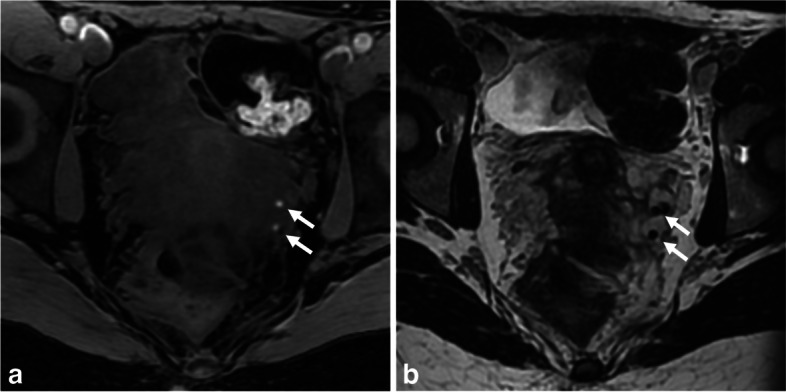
Parametrial left-sided phlebolith in an 18-year-old woman. a Axial T1-W and (b) axial T2-W MR images show a punctiform T1-hyperintense “spot” matching a T2 “dark spot” within the lumen of a parametrial vein (arrows)
